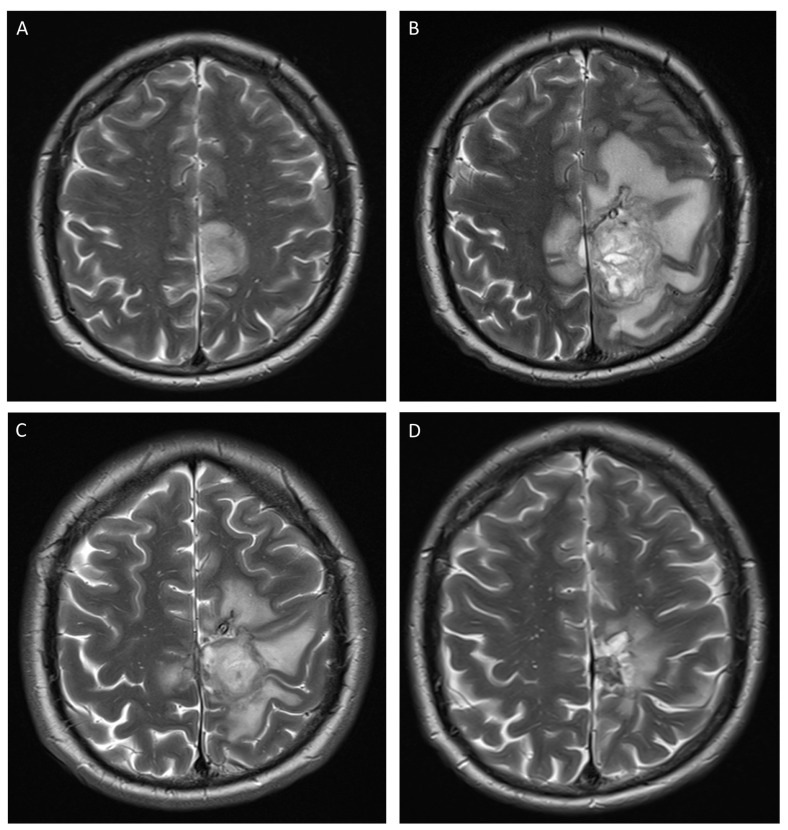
Serial T2-weighted MRI images. (A): Day 1 of admission. Hyperintense lesion with maximal diameter of 35 mm in the left frontal lobe with limited extension to the right frontal lobe. (B): Day 22 of admission. Increased lesion with a maximal diameter of 55 mm and important perilesional edema with small midline shift. (C): Day 70 since hospitalization, six weeks after start of intralesional liposomal amphotericin B. Decreased lesion with a maximal diameter of 43 mm and reduced perilesional edema without midline shift. Ommaya drain visible within the lesion. (D): Nine months since hospitalization. Small residual lesion with maximal diameter of 14 mm, with hemosiderin depositions and surrounding gliosis.
